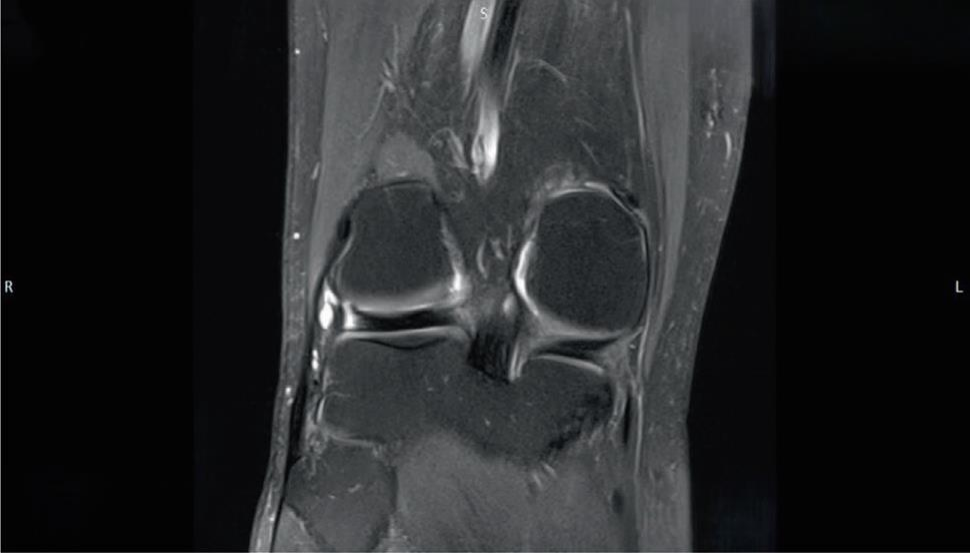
MRI of the right knee joint (coronal view) showing a stable ganglion at the origin and along the course of the popliteus tendon, with a point of maximum prominence at the level of the lateral femorotibial joint line. In this imaging the "R" stands for "right side" and the "L" stands for "left side."
